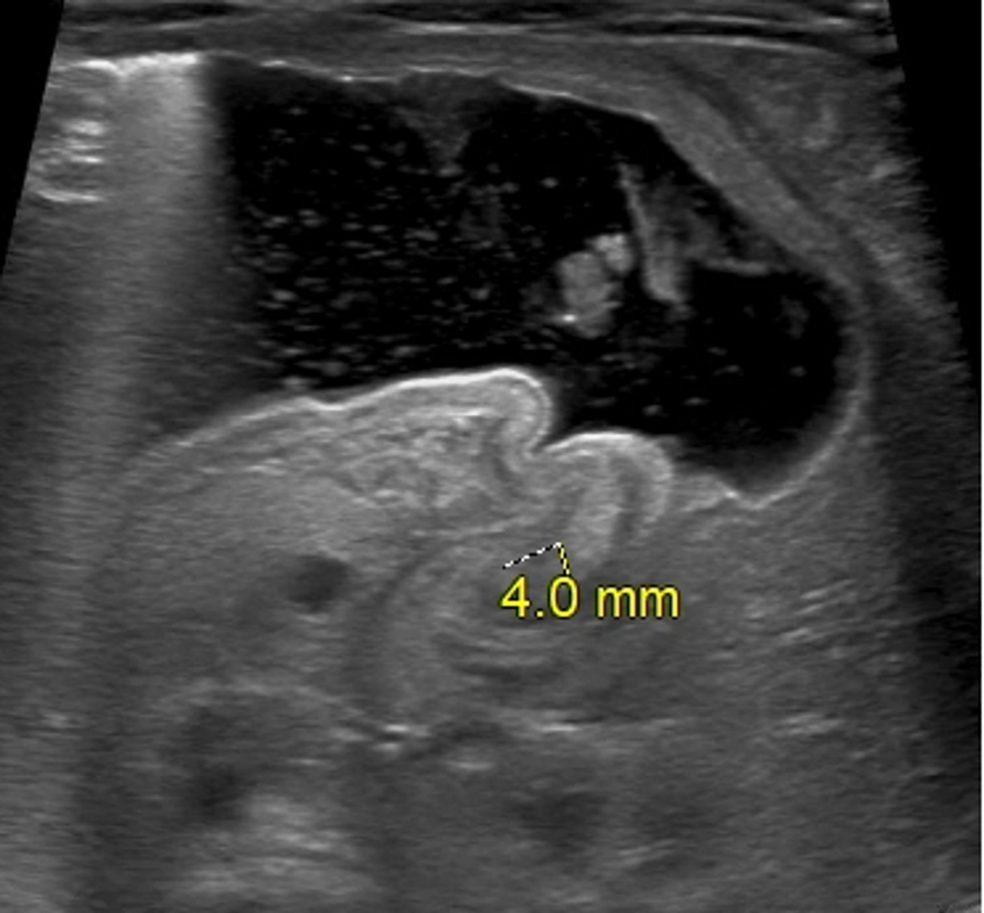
Transabdominal ultrasound revealing pyloric channel thickness measuring 4 mm as represented by yellow dotted line.
